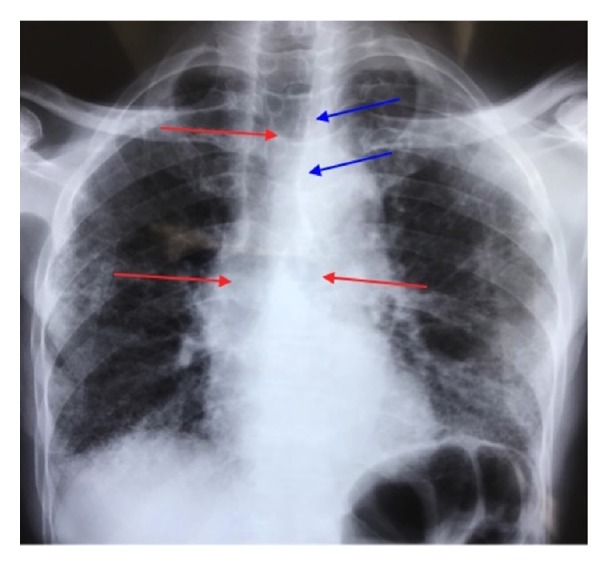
Chest X-ray showing tracheal and proximal bronchi dilation (red arrows) and pneumomediastinum (blue arrows).
